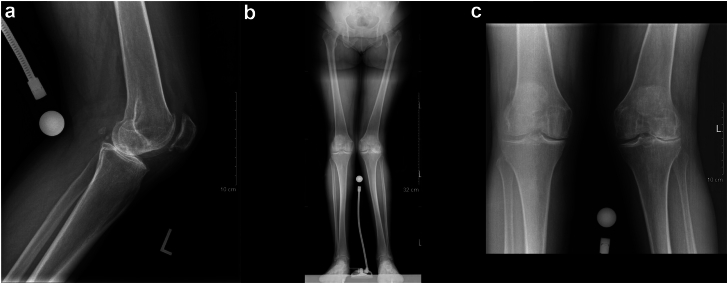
Preoperative X-rays of (a) lateral of the left knee, (b) full-length standing antero-posterior of both lower extremities (c) antero-posterior view of both knees.
